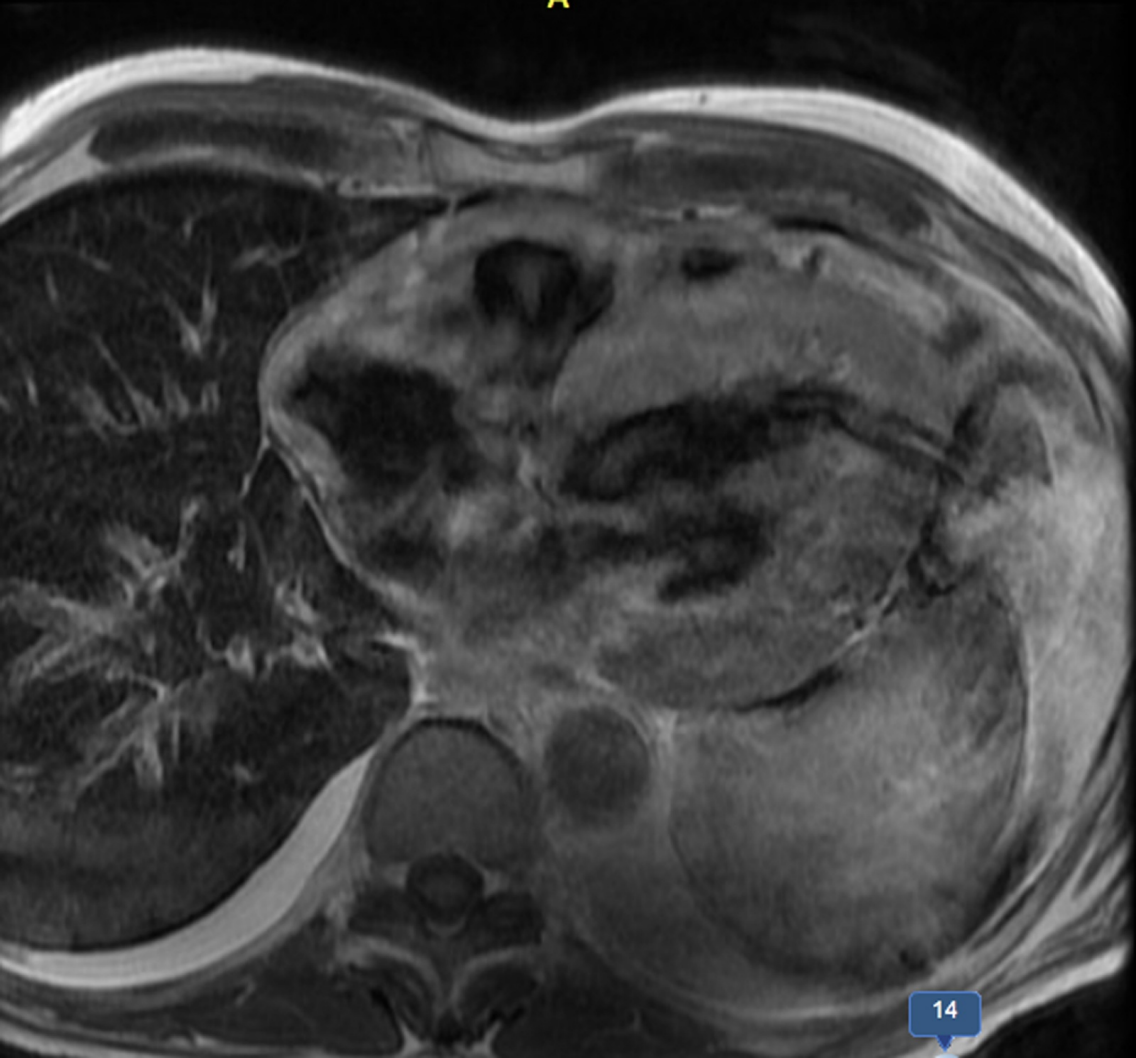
Cardiac MRI with late gadolinium enhancement Diffusely enlarged concentrically thickened ventricles with increased myocardial signal suggestive of amyloid deposition by appearance
